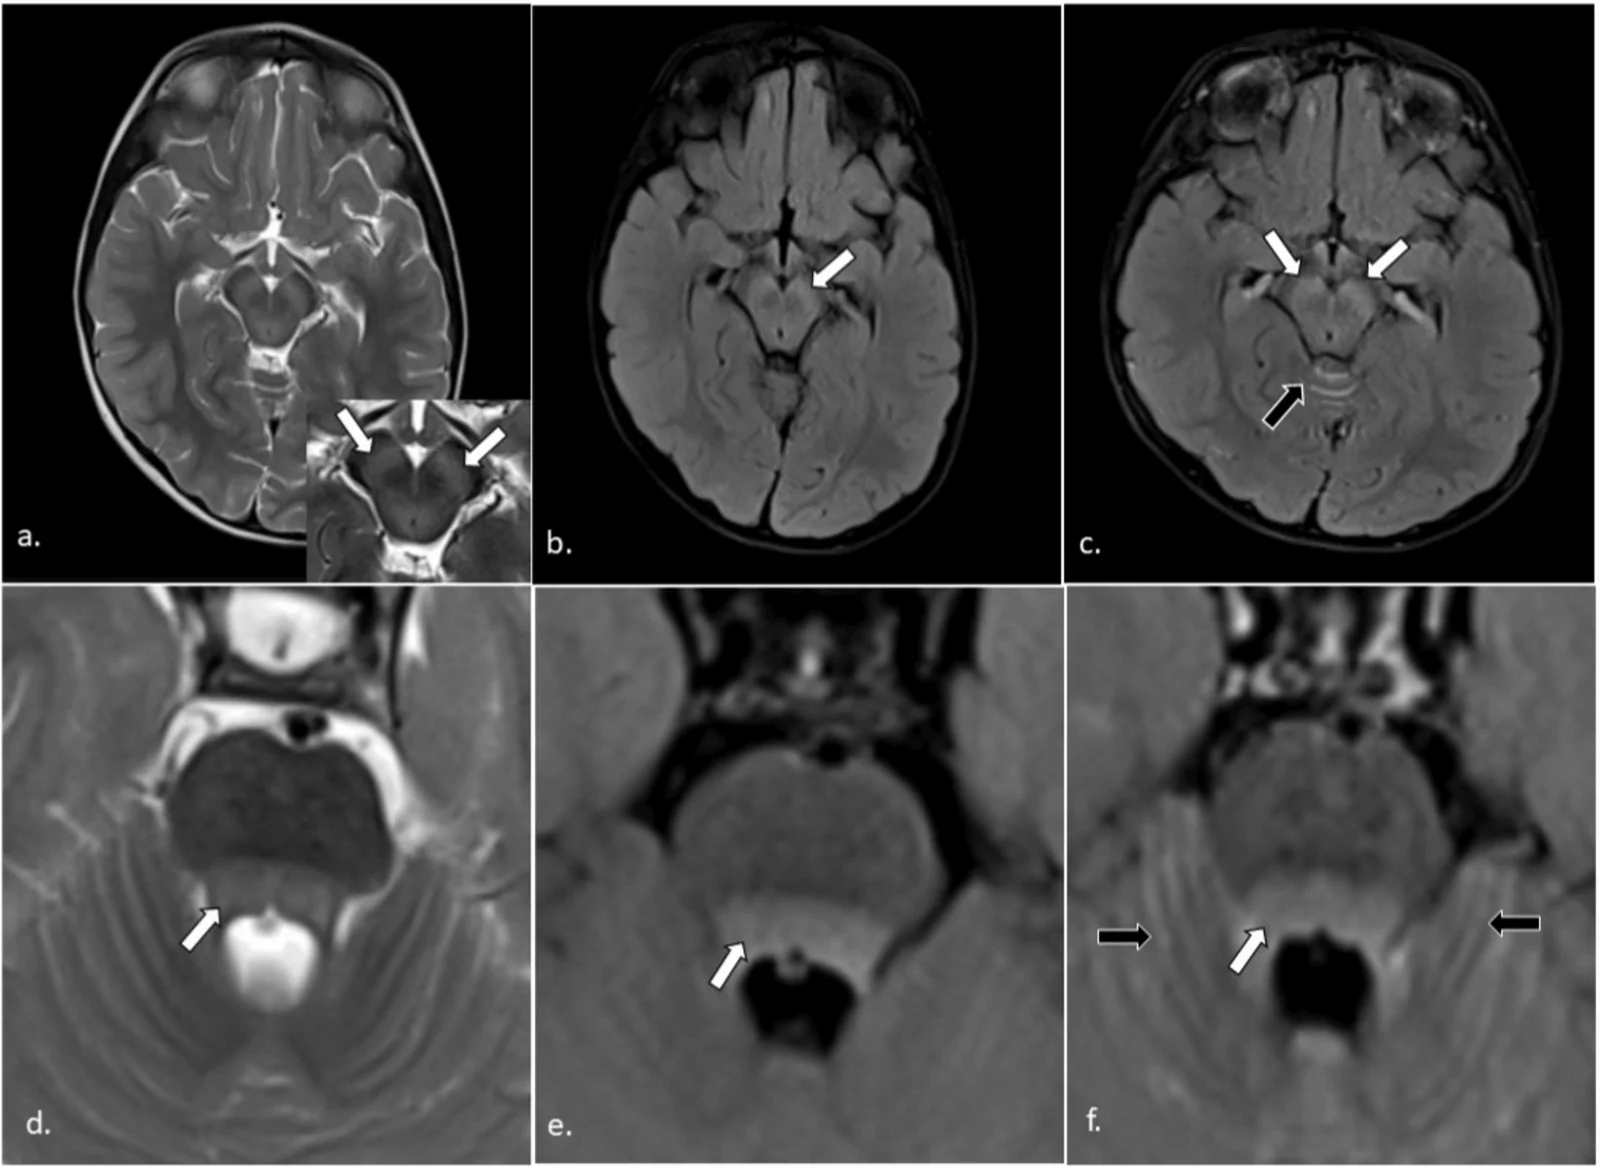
Magnetic resonance imaging of the brain. Axial T2-weighted (T2W) (a), pre-contrast fluid-attenuated inversion recovery (FLAIR) (b), and post-contrast FLAIR (c) images at the level of the midbrain showing hyperintensity involving the bilateral cerebral peduncles with enhancement (arrows) and leptomeningeal enhancement along the vermis (black arrow). Axial T2W (d), pre-contrast FLAIR (e), and post-contrast FLAIR (f) at the level of the pons showing hyperintensity involving the dorsal pons with enhancement( arrows) along the cerebellar folia (black arrows).
